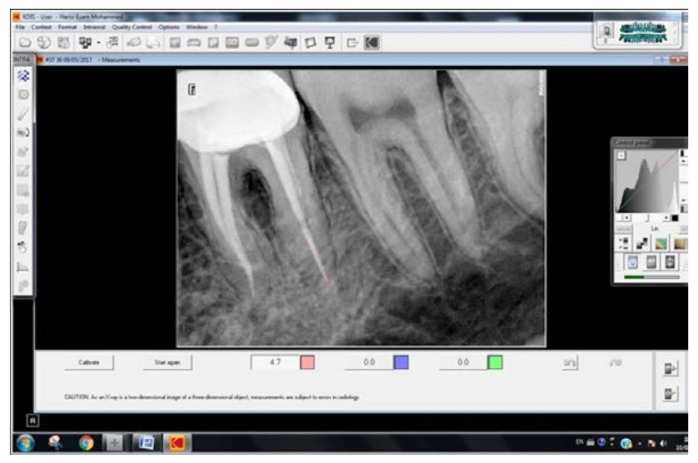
A peri-apical radiograph showing the measurement of gap between the cemented post and remaining gutta-percha filling using the CS-R4 program.
